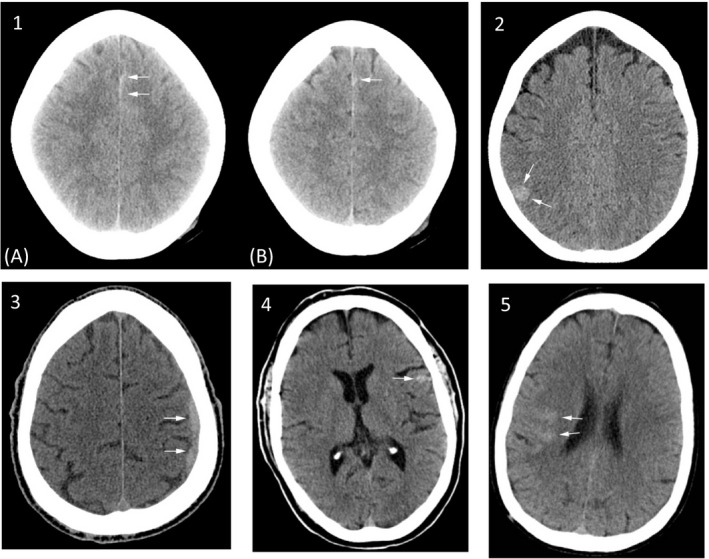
Head CT images of false‐negative subjects. Noncontrast head CT images of five false‐negative subjects. Subject 1: (A) and (B) show focal subarachnoid hemorrhage in the anterior, paramedian frontal sulci. Subject 2: Focal area of hyperdensity in the posterior right parietal lobe, suggestive of a focal hemorrhagic cortical contusion, which was subsequently found on MRI to represent a cavernous malformation. On lower slices (not shown), there is a suggestion of some lower attenuation edema which marginates the contusion. Subject 3: Subdural hemorrhage along the left lateral hemisphere, overlying the frontal and parietal lobes with minimal local mass effect on the brain parenchyma. Subject 4: Left frontal subarachnoid hemorrhage. Subject 5: Right temporal subarachnoid hemorrhage (images for subjects 1–3 reprinted with permission from *Lancet Neurology*)
